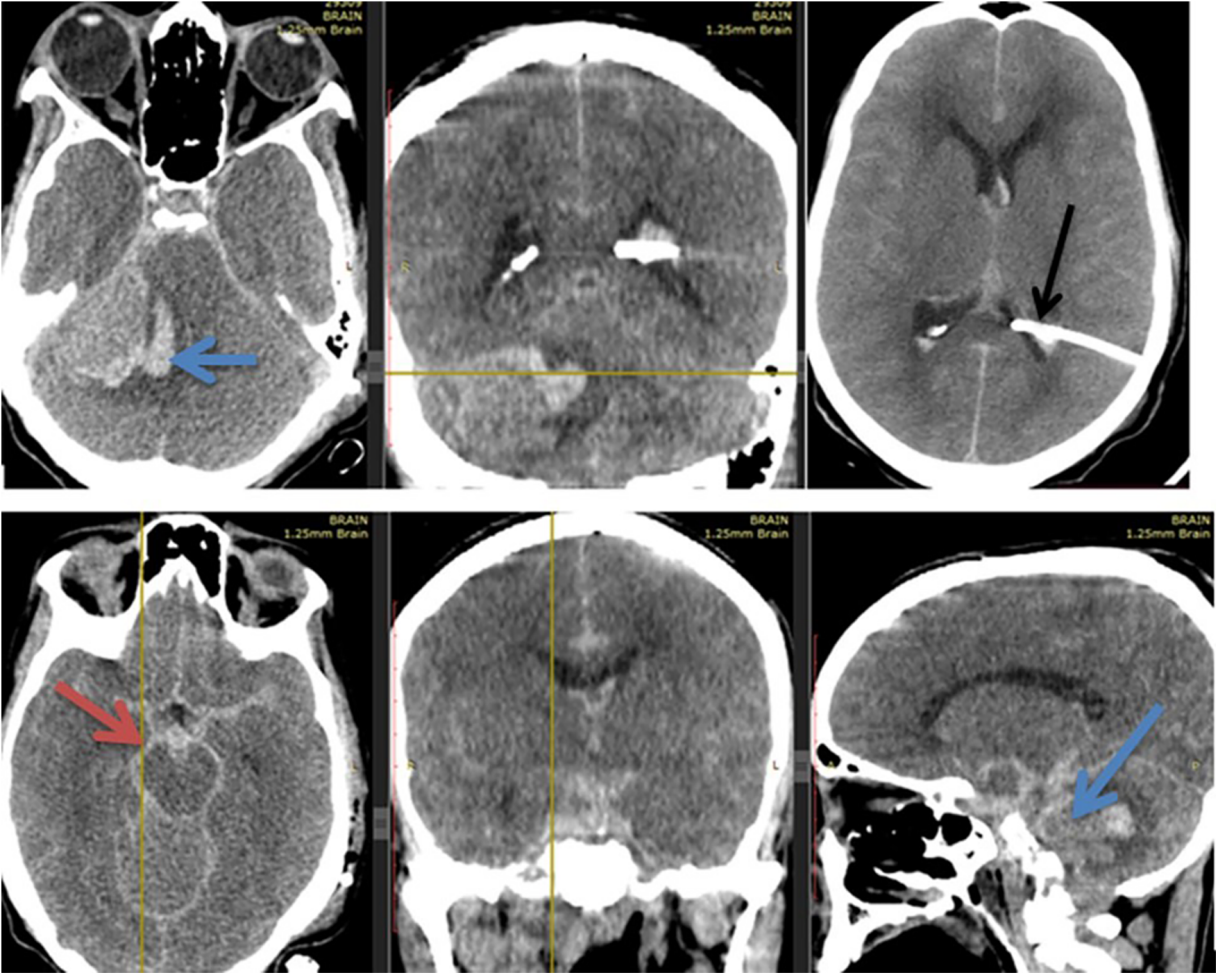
Shows the control CT scan of the patient immediately after neurologic deterioration and showed diffuse basal cistern thick subarachnoid hemorrhage with effaced basal cisterns (marked by the dark red arrow), minimal intraventricular hemorrhage and intra & peritumoral bleeding (as depicted by the dark blue arrow). The proximal shunt tip catheter was inside the left atrium (marked by the dark arrow). There was no malposition & signs of over drainage.
